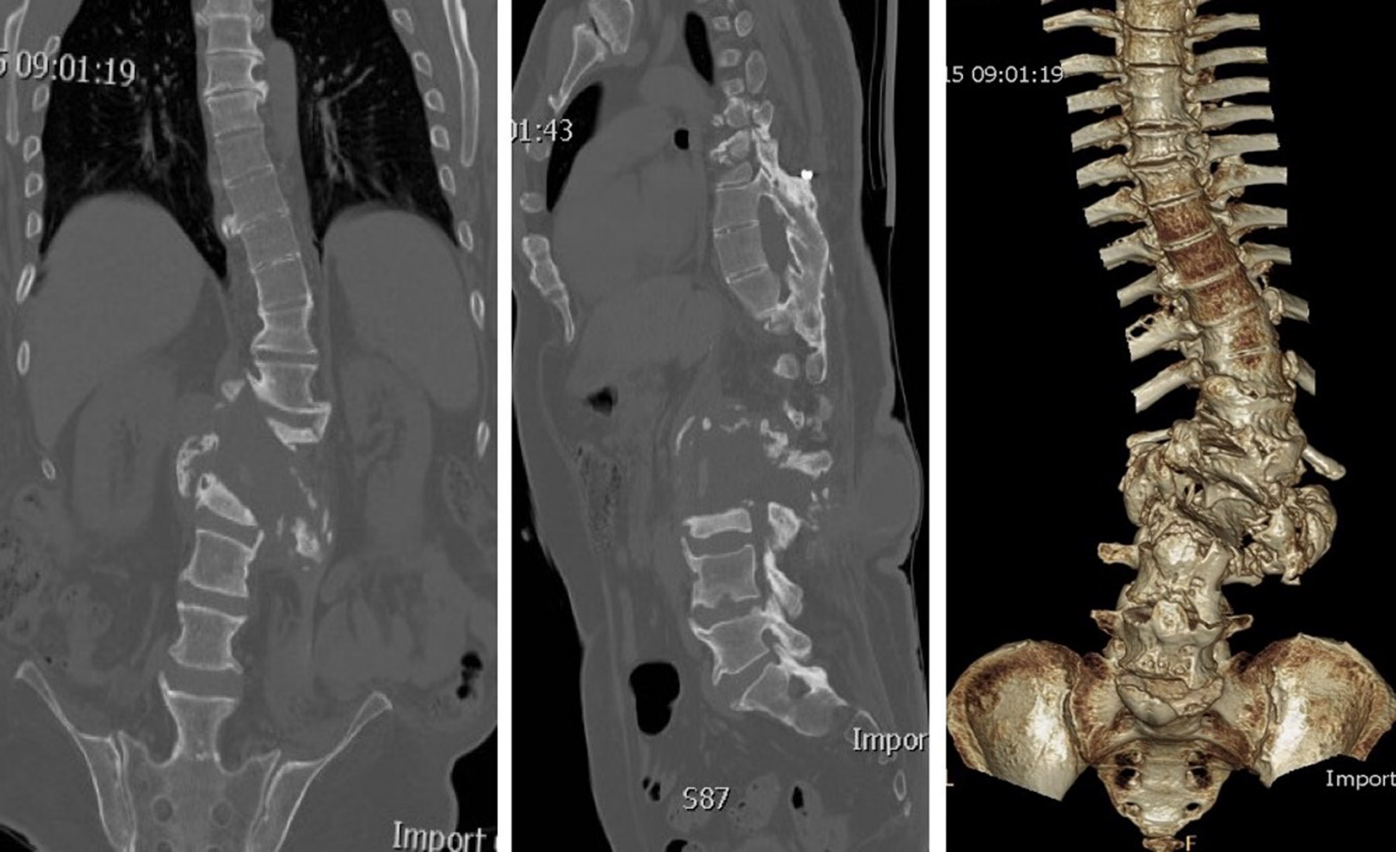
CT scan at presentation showing bony destruction and para-vertebral new bone formation.
